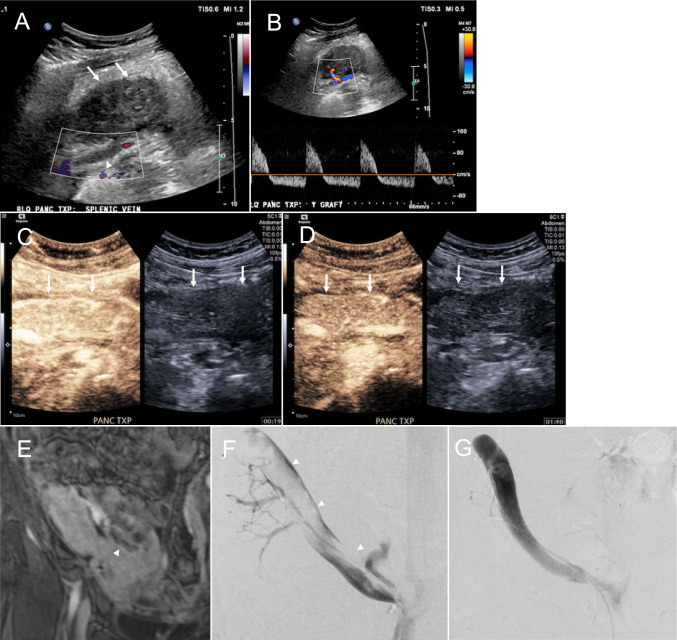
Venous thrombosis. A 44-year-old female with a history of type 1 diabetes mellitus underwent pancreas transplant alone with clinical presentation of increased blood in the urine on postoperative day 1. Color Doppler ultrasound (A) shows a heterogeneous appearing and enlarged pancreas graft (arrow) with no color flow within the graft vein due to thrombosis (arrowhead). Spectral Doppler ultrasound (B) of the intraparenchymal artery shows reversed diastolic arterial waveforms. Contrast-enhanced ultrasound demonstrates prompt parenchymal enhancement at 19 s after injection (arrow, C) and minimal washout at 100 s after injection (arrow, D). Magnetic resonance angiography (E) during venous phase confirms the presence of thrombus in the splenic vein (arrowhead). Pre-treatment venography (F) shows a filling defect corresponding to splenic vein thrombus (arrowhead). Post-treatment venography (G) shows resolution of the thrombus and restoration of venous blood flow
